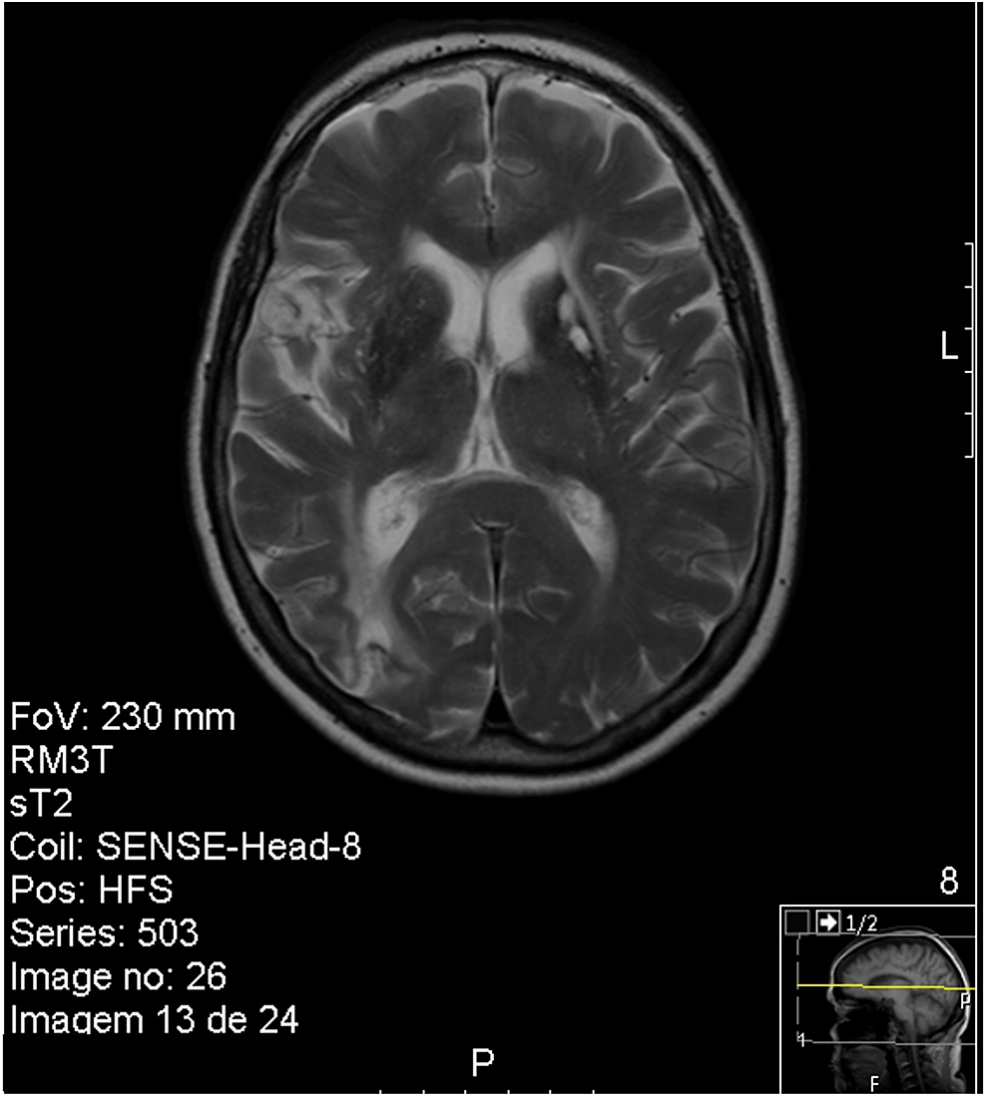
Brain magnetic resonance imaging (FLAIR) reveals multiple hyperintense cortico-subcortical lesions, frontal, parietal, and in the right occipital temporal transition. FLAIR: fluid-attenuated inversion recovery
